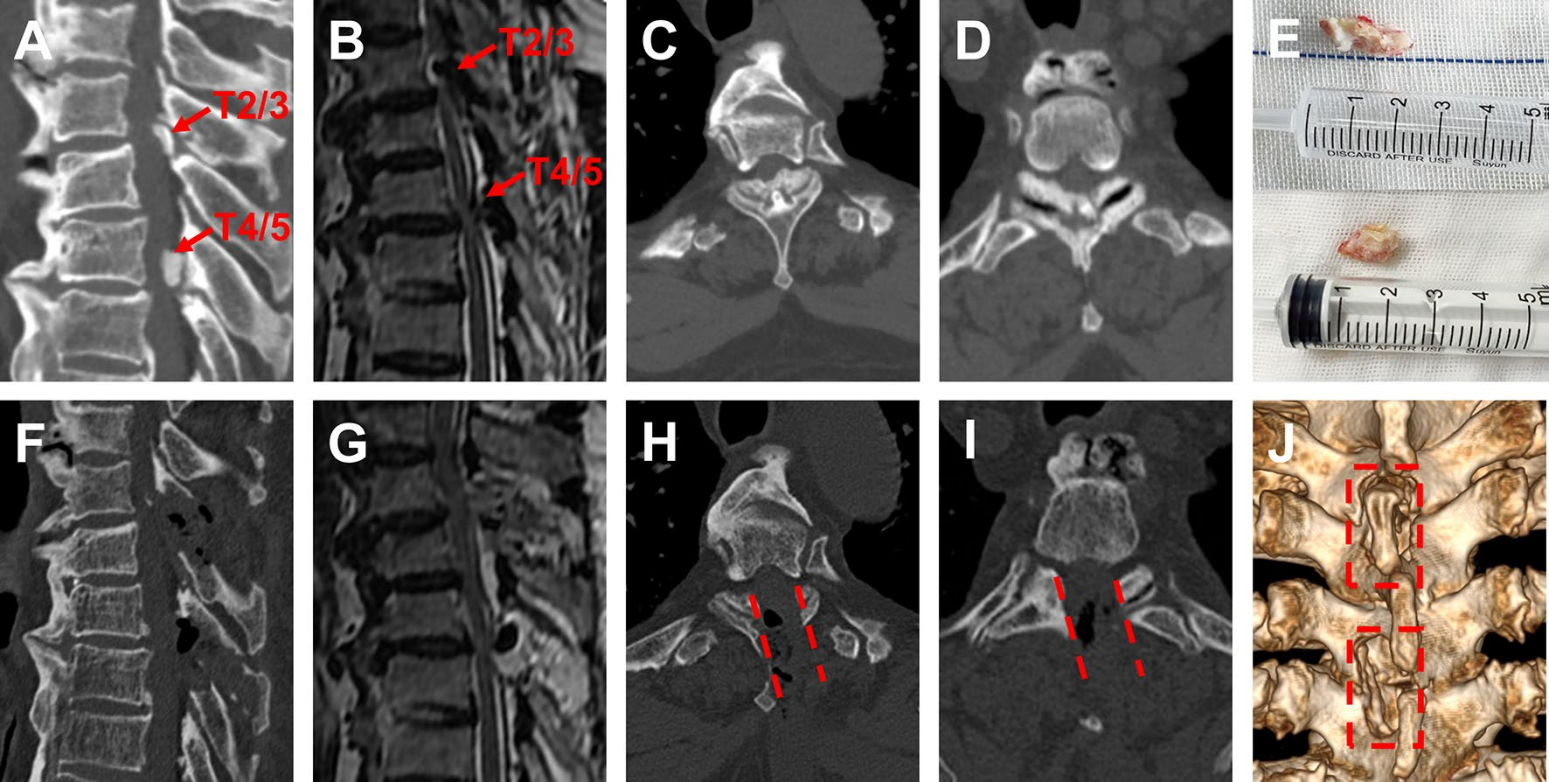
Two segments TOLF treated with OSE procedure. (A–D) Preoperative sagittal, axial CT and MRI images of TOLF, and the ossification lesion compressed the spinal cord at T2/3 and T4/5. Long T2 signal in spinal cord on MRI (As shown by the red arrow). (E) Remove the ossification lesions of T2/3 and T4/5 in piece. (F–I) Postoperative sagittal, axial CT and MRI images, demonstrated a complete remove of ossification lesion (As shown by the red dashed) and good decompression of spinal cord at T2/3 and T4/5. (J) Postoperative 3D-CT image showed the range of unilateral laminectomy at T2/3 and T4/5 (as shown in the red dashed region), bilateral decompression of spinal cord through unilateral approach.
